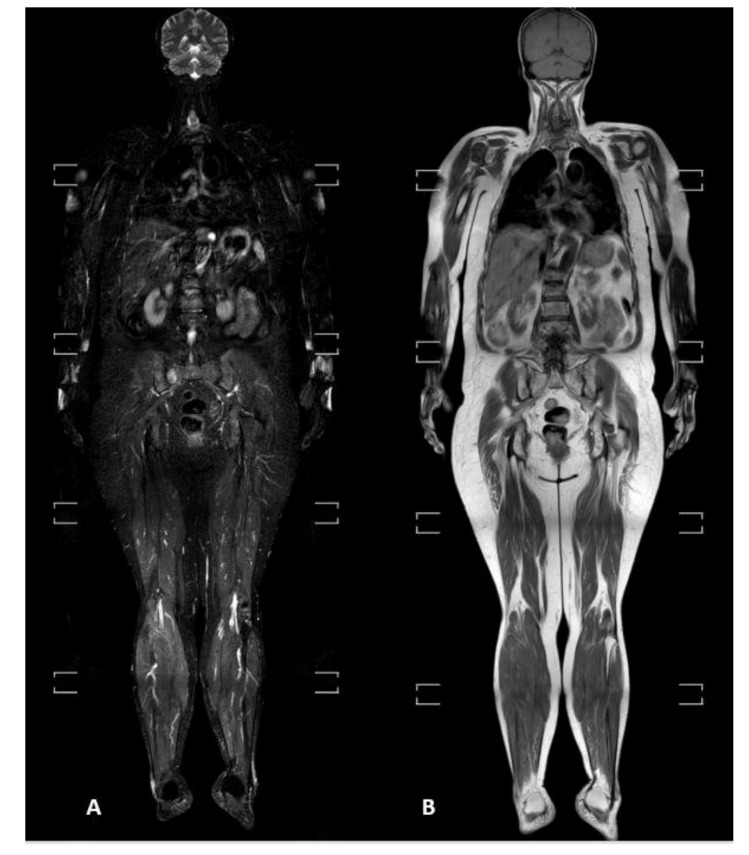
(A) T2-weighted short-tau inversion recovery (STIR) and (B) T1-weighted turbo spin-echo sequences acquired on the coronal plane from the skull vertex to feet.
